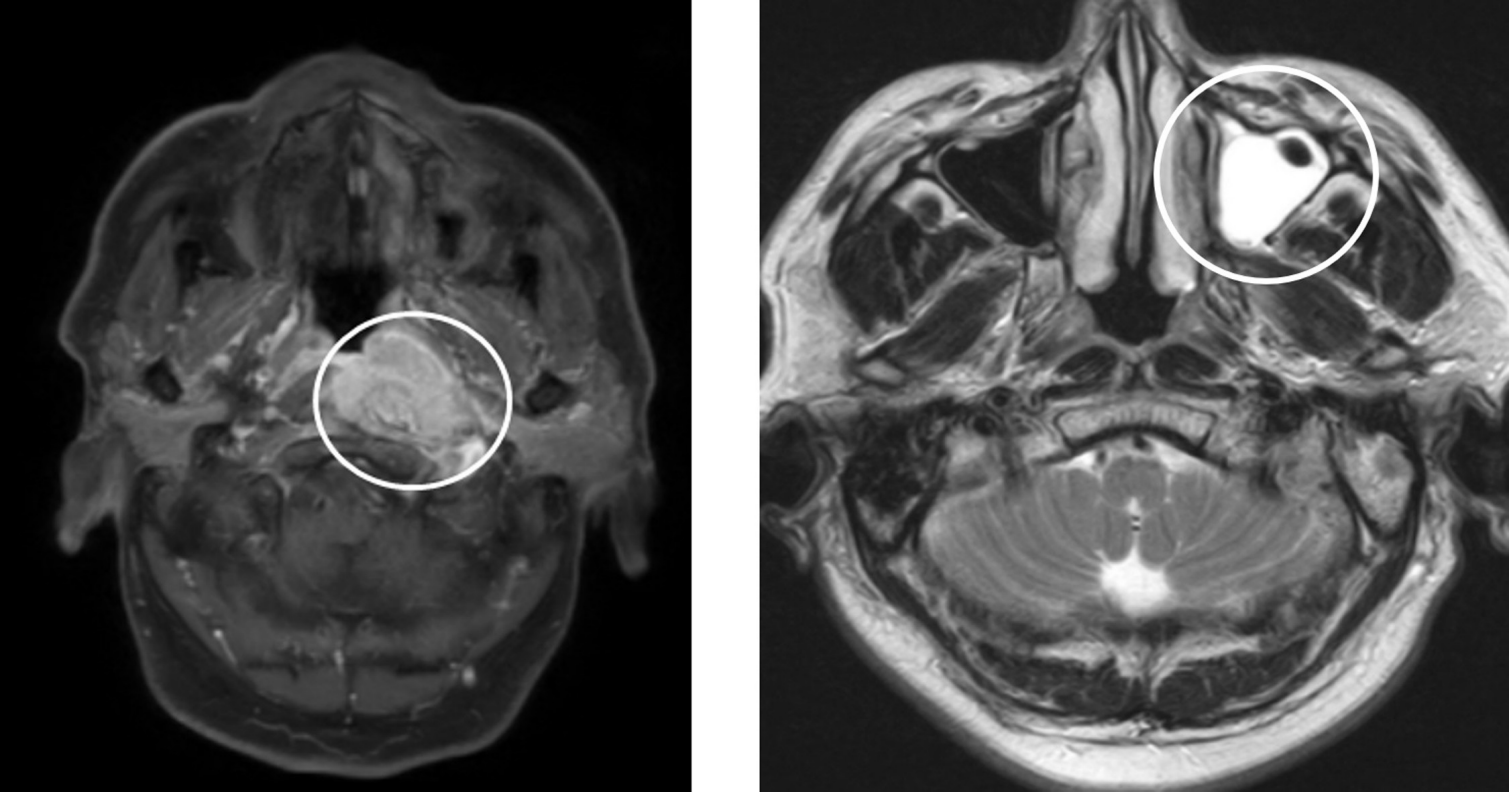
The MRI images. (a) a Stage II NPC patient (b) a CRS patient.
